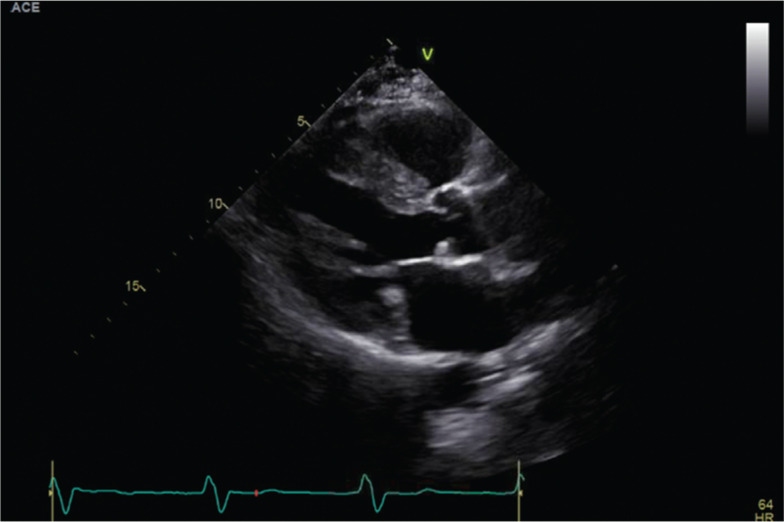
Echocardiogram before device implant.
